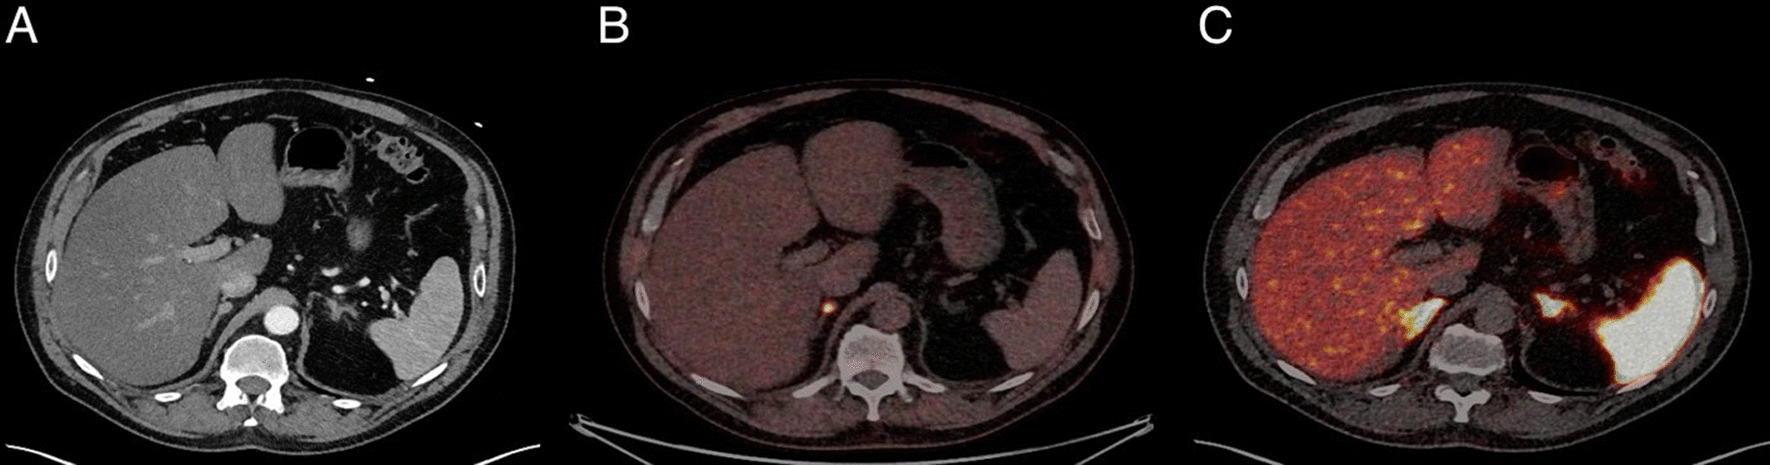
Clinical case presenting a patient suspicious for PPGL. The patient had a history of hypertension. Preoperative normetanephrines were 1.93 and 1.59 (< 1.07 nmol/L) and metanephrines 0.23 and 0.23 (< 0.45 nmol/L). Pathology confirmed a 15-mm pheochromocytoma with a PASS score of 7. The images show transaxial slides of the adrenal glands on a contrast-enhanced CT (left, image A), superimposed on a contrast-enhanced CT for FDOPA PET/CT (middle, image B) and DOTATOC PET/CT (right, image C). Image B shows high, FDOPA uptake in the right adrenal gland correlating to a 15-mm nodular, contrast-enhanced structure (not hypervascular). No FDOPA uptake in the left adrenal gland and no CT correlate. Image C shows the high physiologic uptake of DOTATOC in both adrenal glands, as well as physiological uptake in the liver and spleen. Blood pressure and plasma metanephrines were normalized after surgical resection
